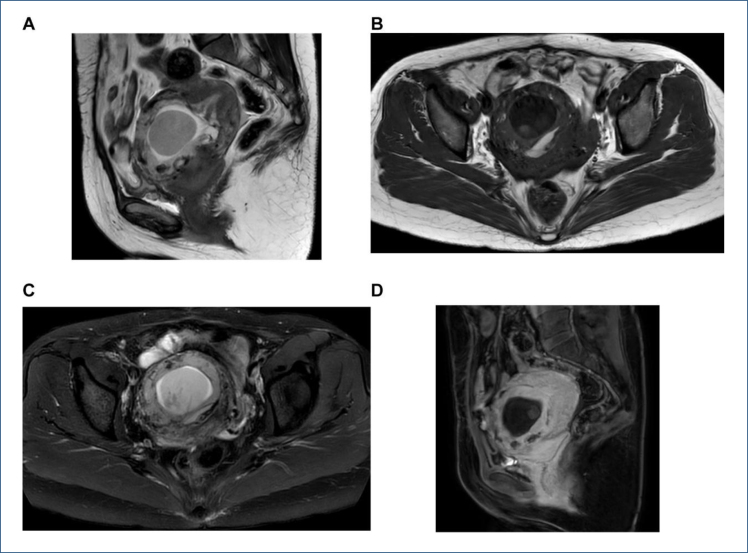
The aforementioned patient T2-weighted image (A), T2-weighted image-SPAIR (B), T1-weighted image (C), and Enhancement sequence (D). Magnetic resonance imaging shows a gestational sac within the uterine cavity, with placental implantation at the site of the previous cesarean section incision (as indicated by the red arrow). The placenta invades the myometrium reaching the serosal layer, with evidence of discontinuity. The boundary between the posterior wall of the bladder and the anterior uterine wall is indistinct.
